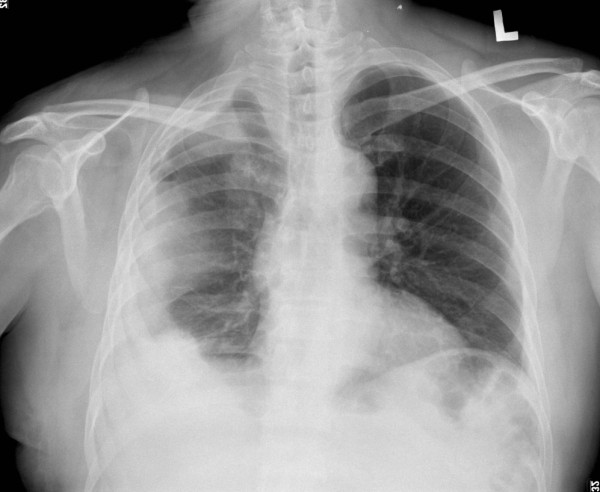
Chest roentgenogram. Plain chest film showed right-side loculated pleural effusion.
